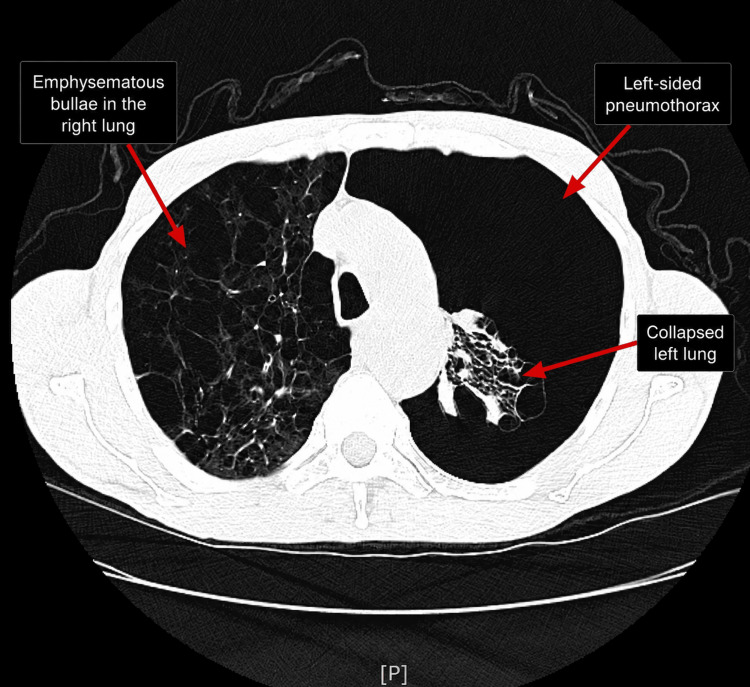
Computed tomography demonstrating a complete left-sided pneumothorax and advanced emphysematous changes Axial computed tomography of the chest obtained before pleural drainage demonstrated a complete left-sided pneumothorax (arrow), with near-total collapse of the left lung (arrow). Advanced emphysematous changes with bullae are visible in the contralateral lung (arrow).
